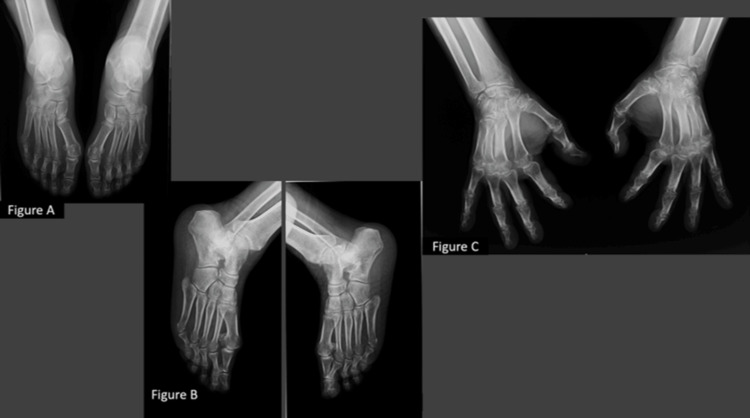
X-rays of the hands and feet X-rays of the hands and feet show reduced periarticular bone density. Figure C also demonstrates soft tissue oedema.
